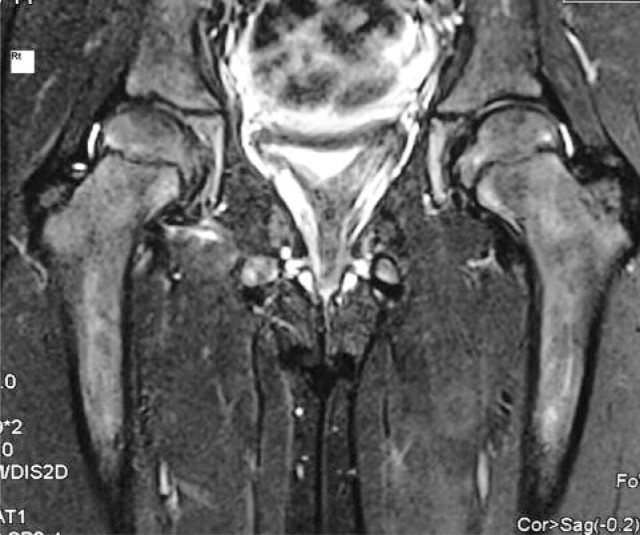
Magnetic resonance image (MRI) of the right hip after one year showed no evidence of avascular necrosis (AVN).
